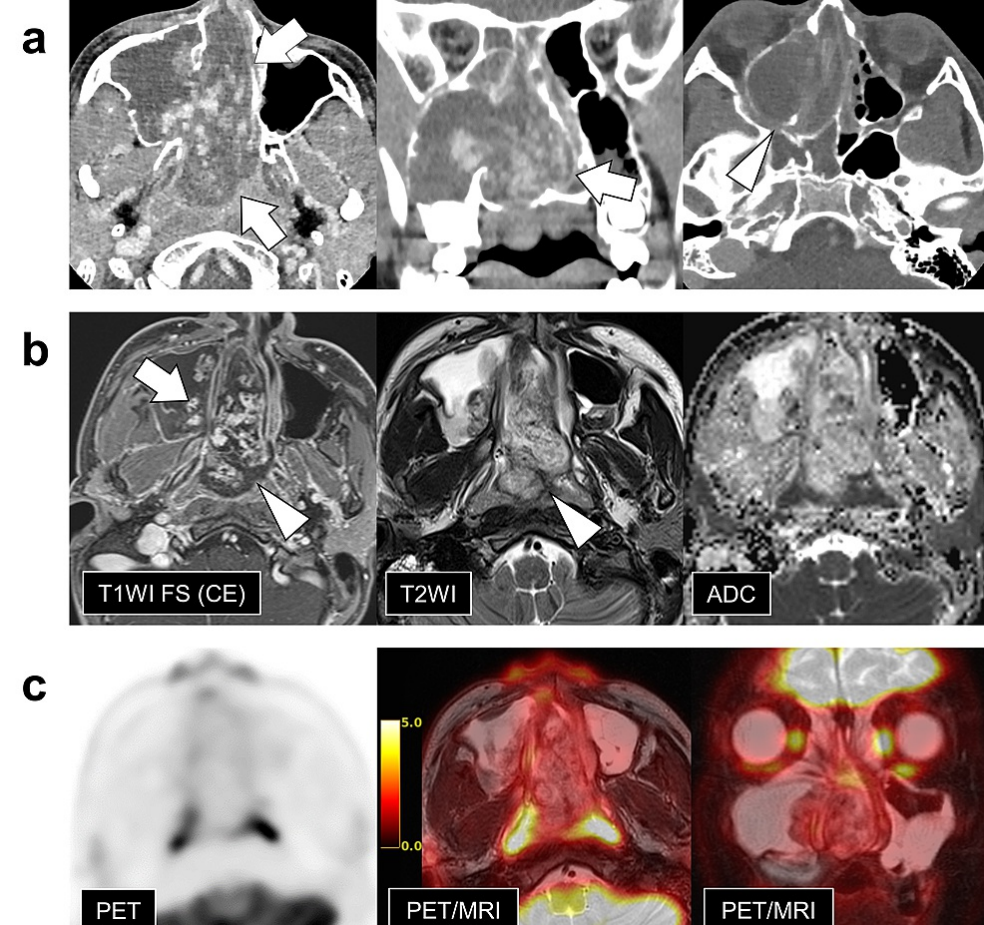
Preoperative imaging findings a) Contrast-enhanced computed tomography (CT), b) contrast-enhanced magnetic resonance imaging (MRI), and c) fused positron emission tomography (PET)/MRI. CT (a) shows a large tumor extending from the right nasal cavity to the nasopharynx (arrows). There is no gross infiltrative bone destruction around the lesion, including the sphenopalatine foramen or pterygopalatine fossa (arrowhead). MRI (b) shows heterogeneously enhanced tumor (white arrow) with unenhanced hypointense rim (arrowheads).The mean apparent diffusion coefficient (ADC) values of the main tumors in the nasal cavity were 2.06×10^-3^ mm^2^/s. PET (c) reveals that the region depicted by T2-weighted imaging shows faint [18F]-2-fluoro-2-deoxy-D-glucose accumulation on PET.
